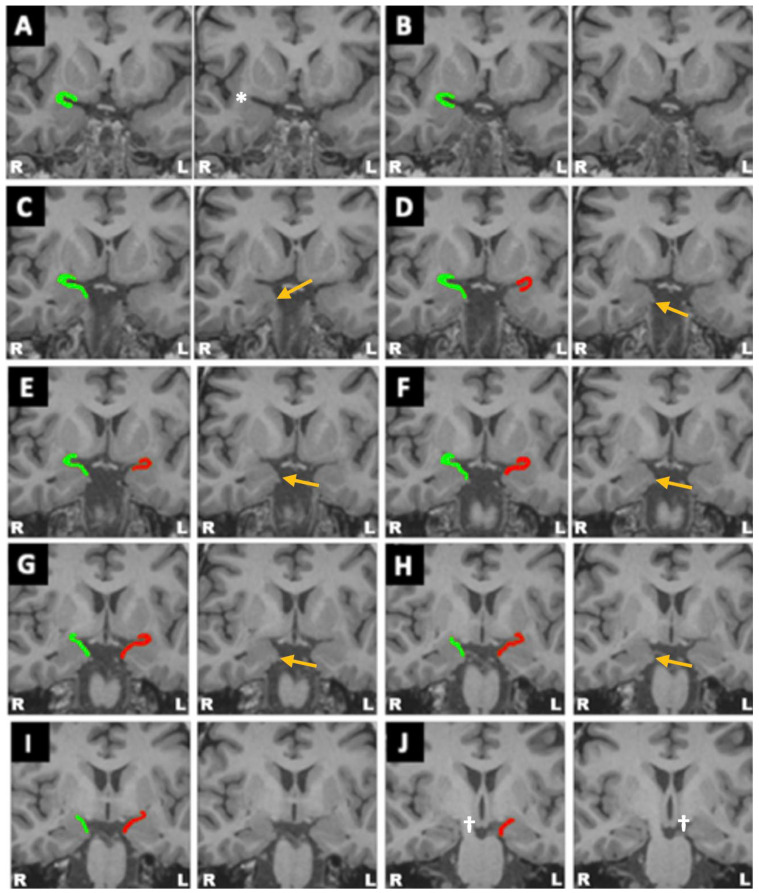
High-resolution MRI showcasing the PC and the relevant anatomical landmarks used to identify it; the right PC is highlighted in green, and the left PC is highlighted in red. Panels (A–J) showcase different coronal planes of showcasing the piriform from its most anterior aspect (A) to its most posterior aspect (J). The orange arrow shows the apex of the gyrus semilunaris (the lower limit of the PC), and the * shows the limen insulae, which marks the beginning of the PC (its anterior limit), while the appearance of the cerebral peduncles, labeled by the cross sign (†), marks the end of the PC (its posterior limit). Taken and modified from a previously published work belonging to the main author under a CC BY 4.0 license [18].
